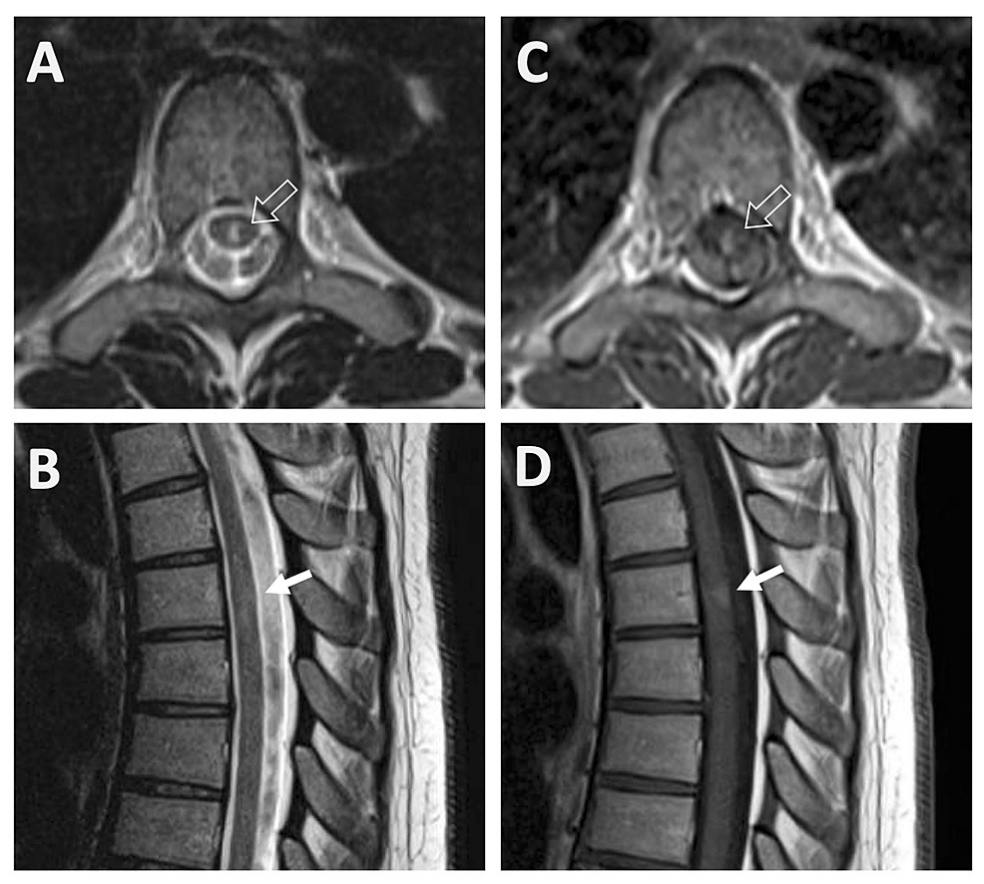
Images (A) and (B): Axial and sagittal T2-weighted sequences showing a centrally located short segment T2 hyperintense cord lesion without associated mass effect. This is less well seen on the sagittal image due to its narrow transverse diameter. Images (C) and (D): Post-gadolinium T1-weighted images showing moderate enhancement of the same lesion.
